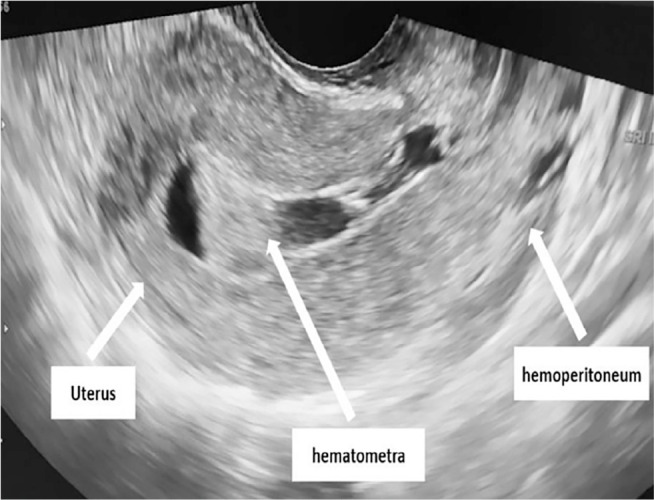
Pelvic ultrasound scan showing a moderate hemoperitoneum and an empty
